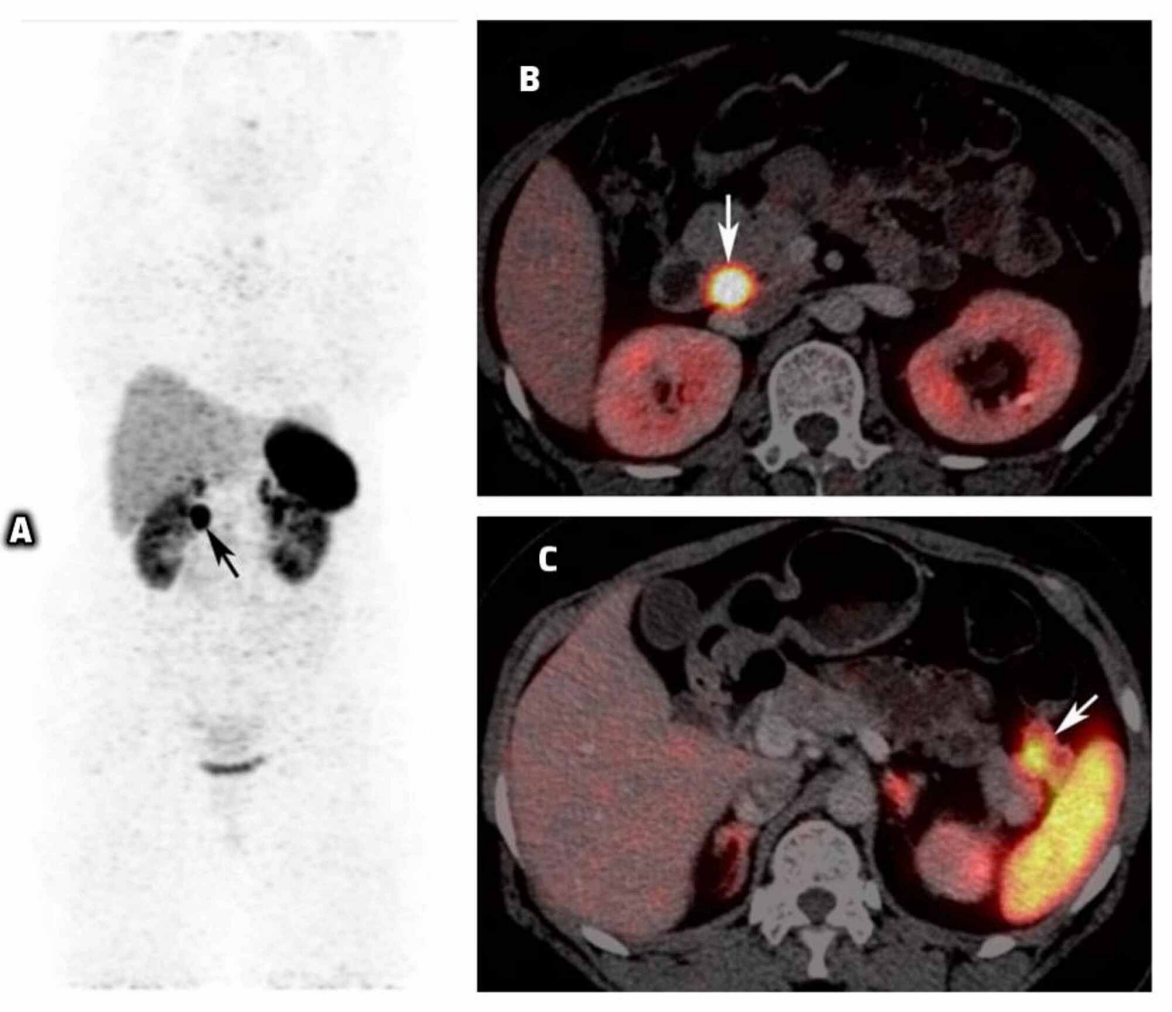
Ga-68-DOTANOC scan images of the patient Maximum intensity projection (MIP) image (A) and axial fused positron emission tomography/computed tomography (PET/CT) images (B and C) show two somatostatin receptor expressing lesions in the pancreatic head (1.6 cm x 1.5 cm) (B) and tail region (3.2 cm x 2.2 cm) (C), respectively.
